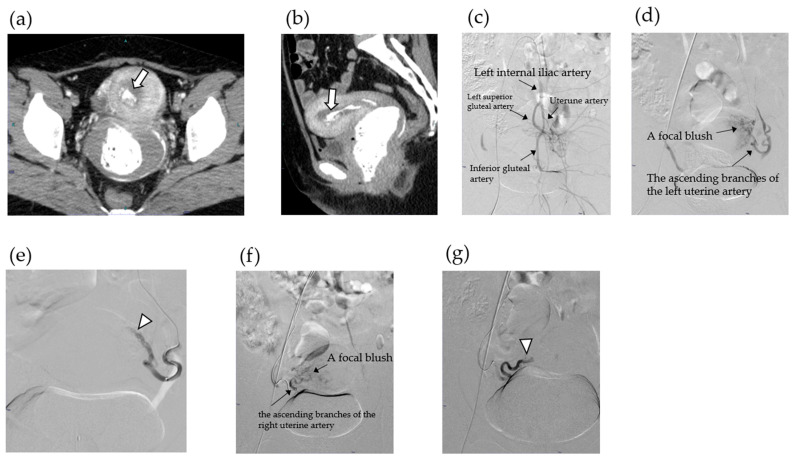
Arterial-phase contrast-enhanced computed tomography showing focal enhancement (arrow) within the endometrium in the axial (a) and sagittal (b) planes. Pelvic aortography showing the anatomy of the pelvic vessels (c) and the focal blush from the ascending branch of the left uterine artery (d). Post-embolization aortography showing contrast stasis without focal blush following complete embolization of the ascending branch of the left uterine artery using a gelatin sponge (arrowhead) (e). Pelvic aortography shows a focal blush from the ascending branch of the right uterine artery (f). Postembolization aortography showing contrast stasis without a focal blush following complete embolization of the ascending branch of the right uterine artery using a gelatin sponge (arrowhead) (g).
